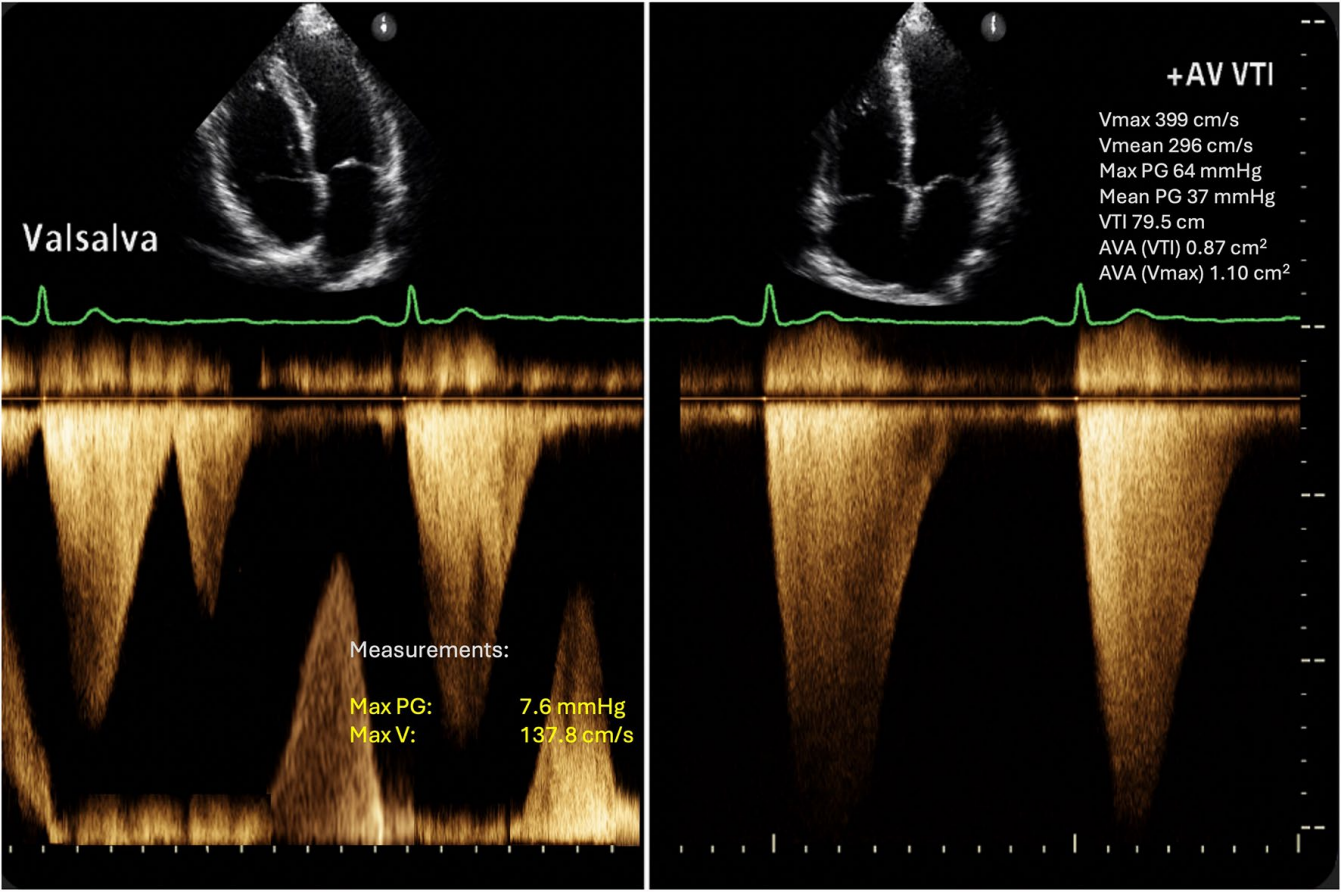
Baseline LVOT spectral Doppler showing late peaking Doppler waveform (left). Transaortic continuous Doppler indicates severe aortic stenosis by peak velocity 399 cm/s
